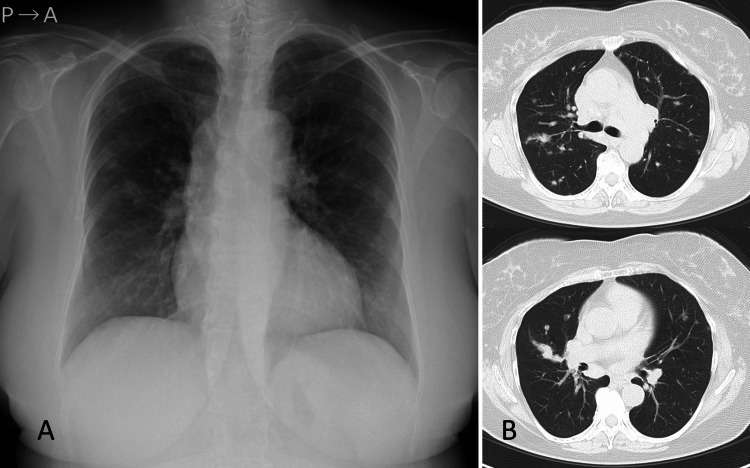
Chest images (A) Chest radiograph showing a new nodular lesion in the middle of the right lung. (B) Computed tomography scan of the chest revealing a new pulmonary nodule (2.5 cm in diameter) within the right superior lobe, multiple smaller nodules that are predominantly distributed in the superior lung fields, and enlarged mediastinal lymph nodes.
